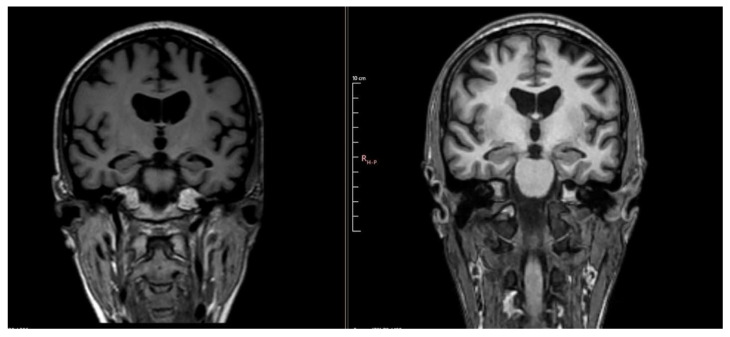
Comparative images showing progression of atrophy between MRI 4 years ago (left) and MRI 1 year ago (right).
